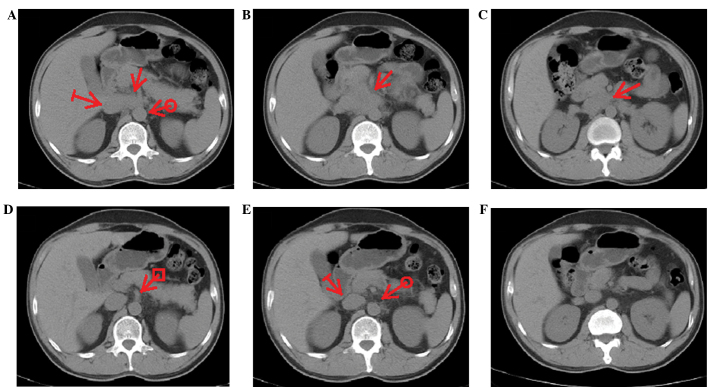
Following administration of docetaxel plus interferon-α for 6 months, a metastatic retroperitoneal lymph node tumor disappeared in the computed tomography scans of a 58-year-old male. (A–C) Pre-treatment image of retroperitoneal lymph node tumor (6 cm in diameter) located between the aorta and vena cava. The boundaries of the tumor and the vena cava are unclear (arrow, metastatic retroperitoneal lymph node; flat-shaped arrow, vena cava; circular arrow, aorta). (D–F) Post-treatment image demonstrates the disappearance of metastatic mass. The vena cava and aorta boundaries are clearly defined (square arrow, superior mesenteric artery).
